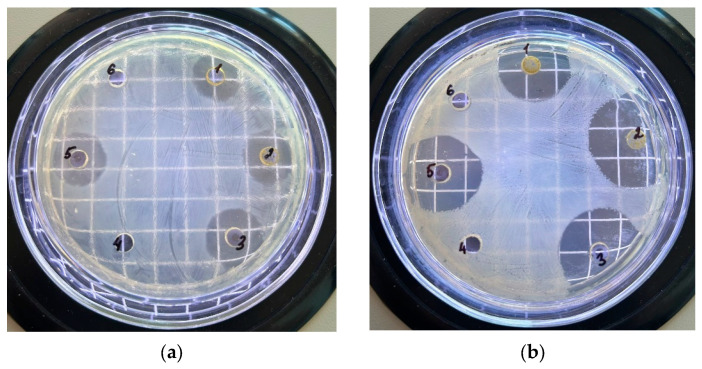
Appearance of the Petri dish field after 24 h of inoculation of *C. michiganensis* (a) and *E. carotovora* (b) cultures when exposed to *G. uliginosum* extract: flowers (1), leaves (2), total biomass (ultrasound treatment) (3), roots (4), total biomass (maceration) (5), stems (6).
